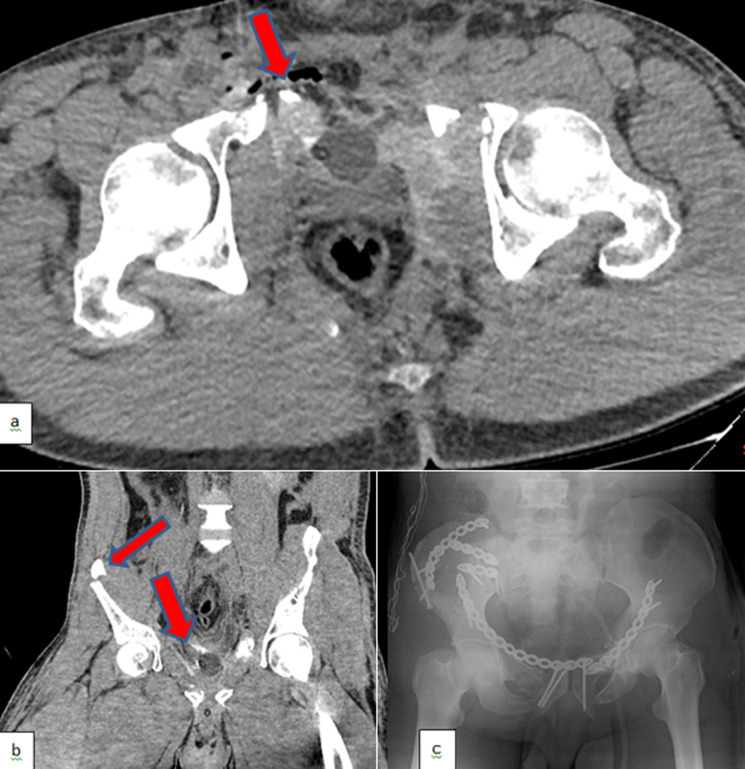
a) axial plain images of computed tomography showing free air and extravasation of contrast from the bladder; b) coronal plain images of computed tomography showing iliac wing fracture and extravasation of contrast from the bladder; c) plain X-ray showing multiple screw fixations and surgical plates (red arrows)
